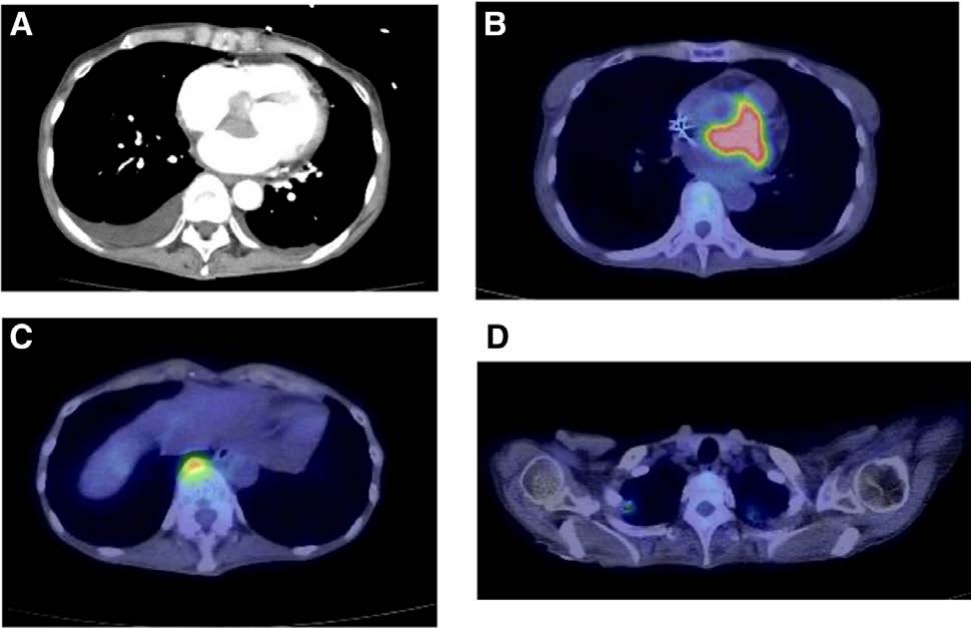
Mass lesion on CT (A). ^18^F-FDG-PET/CT showing uptake in the cardiac mass (B), anterior mass of the twelfth thoracic vertebra (C), and both lung apices (D).
